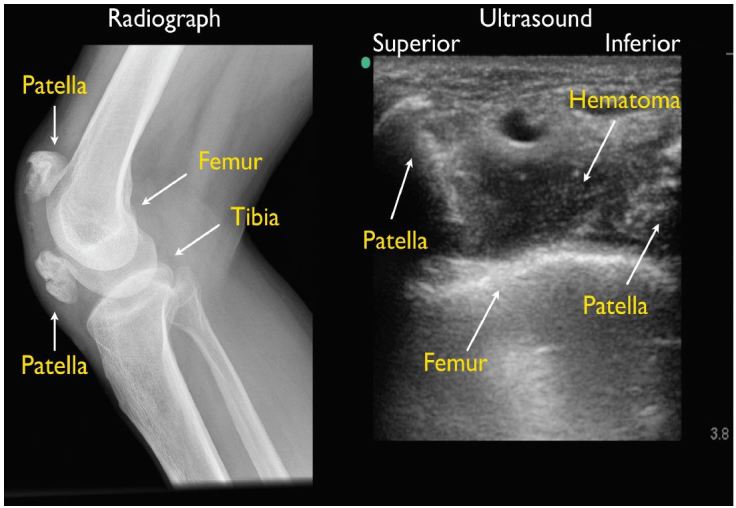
Patient’s knee radiograph and ultrasound.
